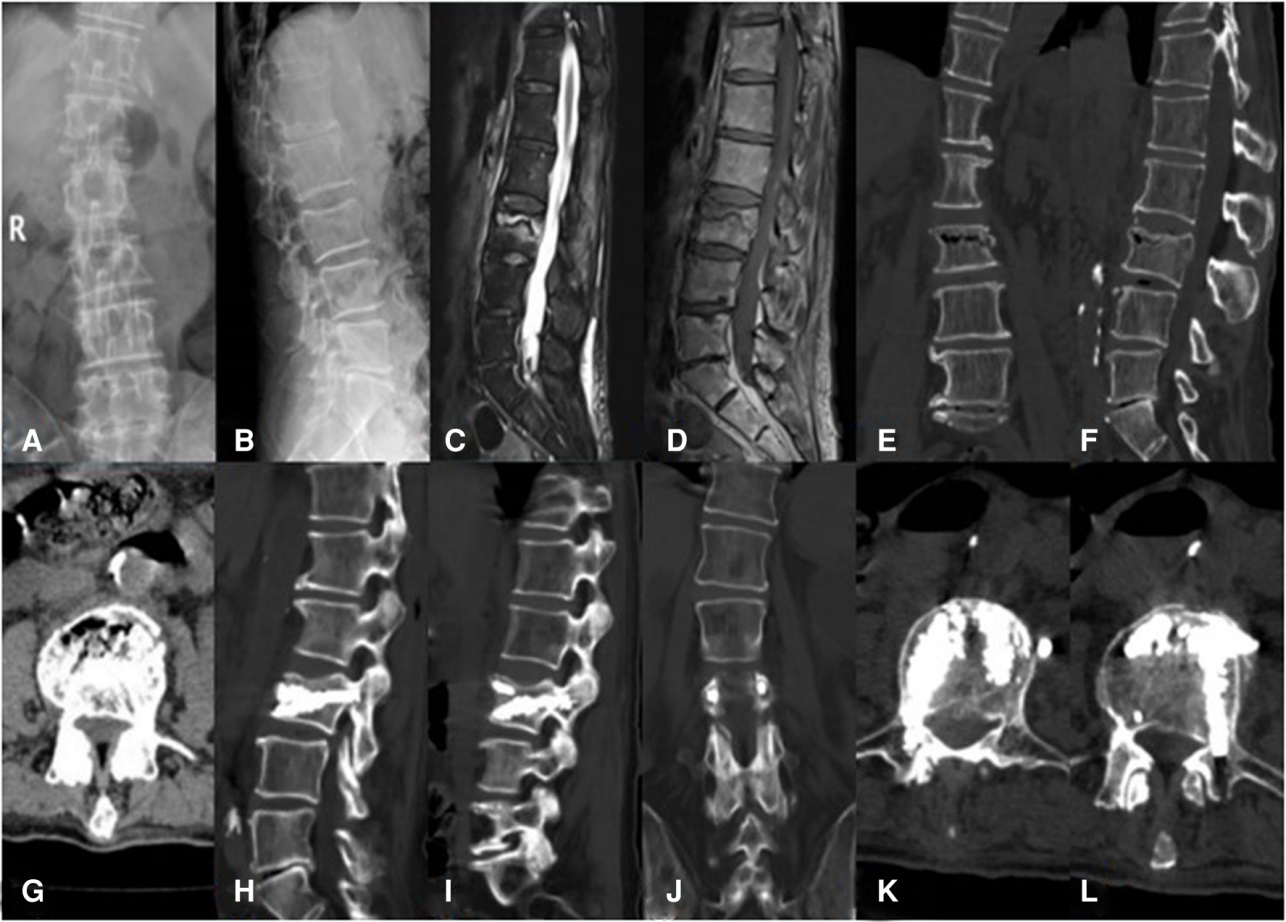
An 81-year-old male patient with KD at L3 treated using bilateral pedicle anchoring technique with PVP: (A,B) preoperative radiographic images; (C) the sagittal T2-weighted MR image showing high signal intensity in the cleft; (D) the sagittal T1-weighted MR image showing low signal intensity in the cleft; (E–G) CT scans showing the intravertebral vacuum sign; (H,I) CT scans after operation showing the bone-cement-filled cleft and vertebrae; (J–L) bone cement dispersion was observed in both pedicles. KD, Kümmell's disease; PVP, percutaneous vertebroplasty.
